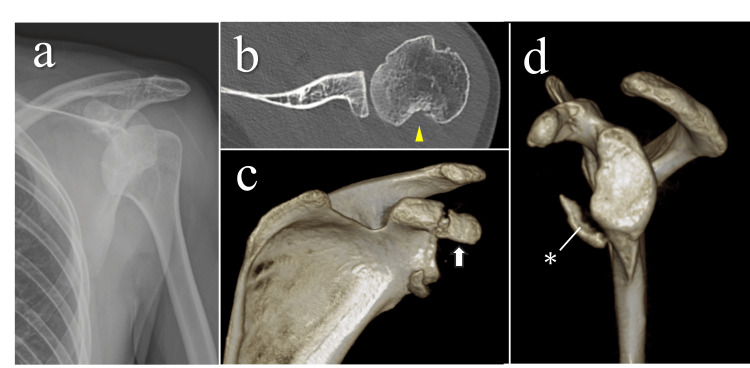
Radiological findings at the time of dislocation and after reduction (a) Anteroposterior radiographs showing anterior shoulder dislocation. (b) Axial CT image demonstrating a Hill-Sachs lesion (20 mm wide and 12 mm deep; yellow arrowhead). (c) Three-dimensional CT image showing a coracoid process fracture classified as Type II according to Ogawa’s classification (white arrow). (d) Three-dimensional CT lateral view showing a bony Bankart lesion involving approximately 20% of the glenoid rim (asterisk). CT, computed tomography
